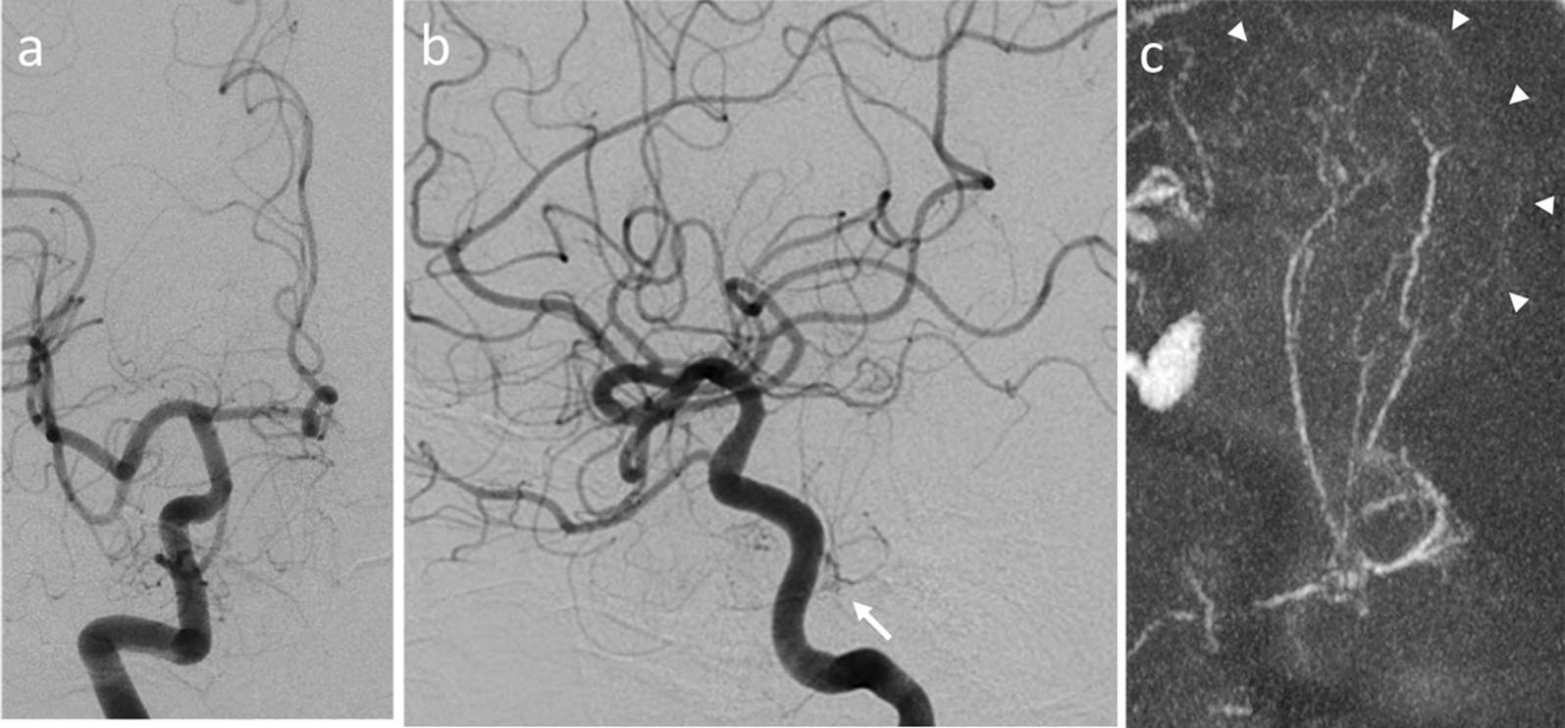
a, b Right internal carotid angiograms, anteroposterior (a) and lateral (b) views, showing the tumor mainly fed by the right meningohypophyseal trunk (*arrow*). c Sagittal maximum-intensity projection cone-beam computed tomography image showing a giant hypervascular pituitary tumor (*arrowheads*) with large feeders from the right meningohypophyseal trunk
